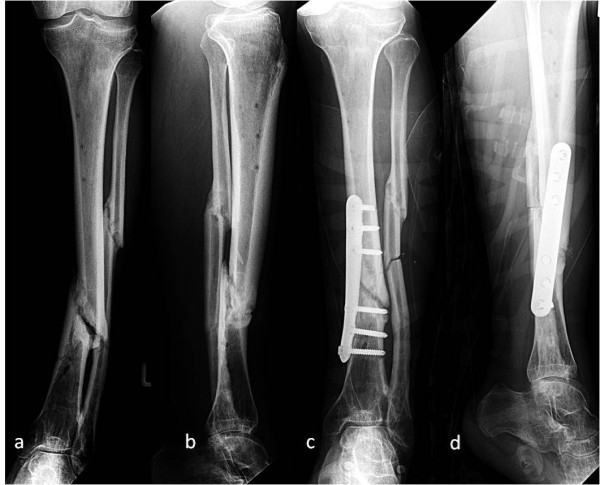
Consecutive x-rays of a 30-year-old male with tibial non-union and valgus malalignment following stabilization with external fixator for 6 months (Figures a,b). Postoperative findings after excision of necrotic tissue and re-osteosynthesis with a multidirectional locking plate (tifix®-tibia-plate, Litos, Hamburg, Germany) in combination with reconstruction of skeletal defects by implantation of autologous bone removed from the iliac crest (Figures c,d). Conventional and molecular bacteria detection methods were both negative.
